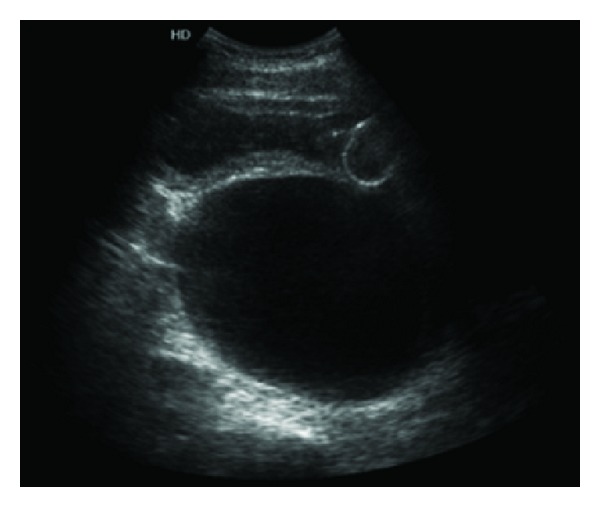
Abdominal ultrasound: large cystic image posterior to bladder with an approximate volume of 780 cc.
